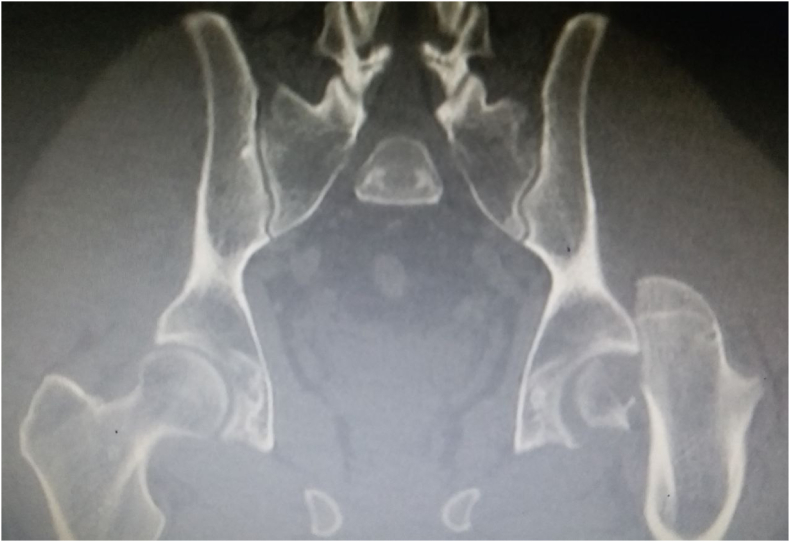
Preoperative CT scan of the pelvis shows the dislocation with the fracture of the femoral head, Pipkin fracture type 1.
